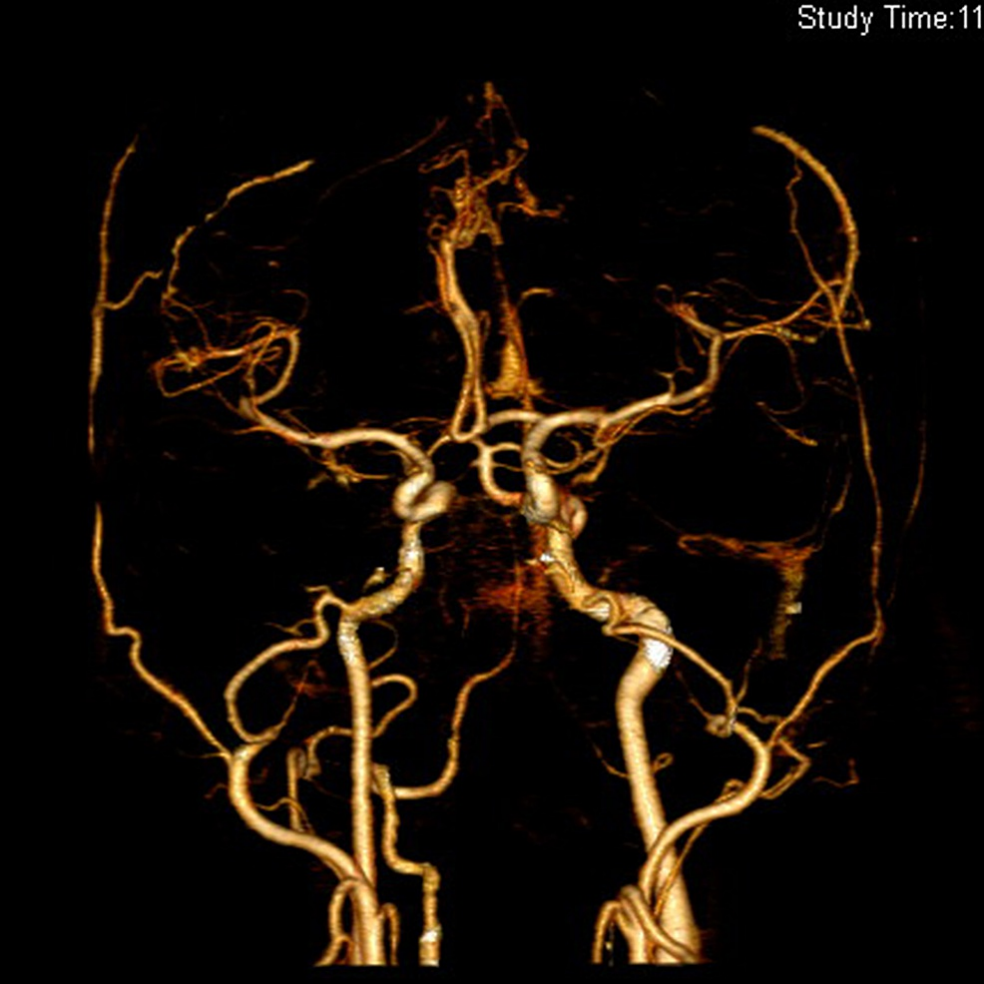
Cerebral CTA performed one year after discharge revealed no aneurysm CTA: computed tomographic angiography
